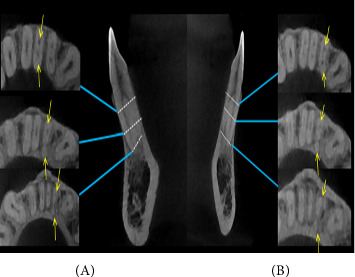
(A, B) CBCT images of two mandibular lateral incisors with two roots. “Dots” represent the axial views of root canal at different levels coronal third, mid-root, and apical third. “Yellow arrow” pointing.
